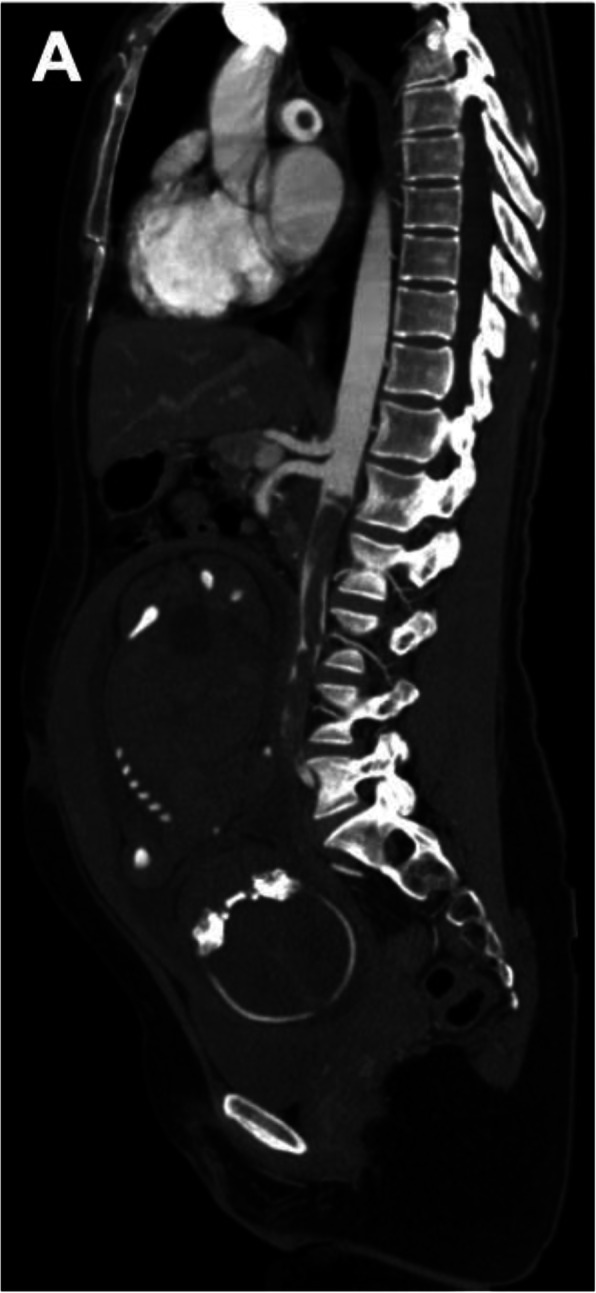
Angio-CT - sagittal view - showing juxta renal aortic thrombosis
